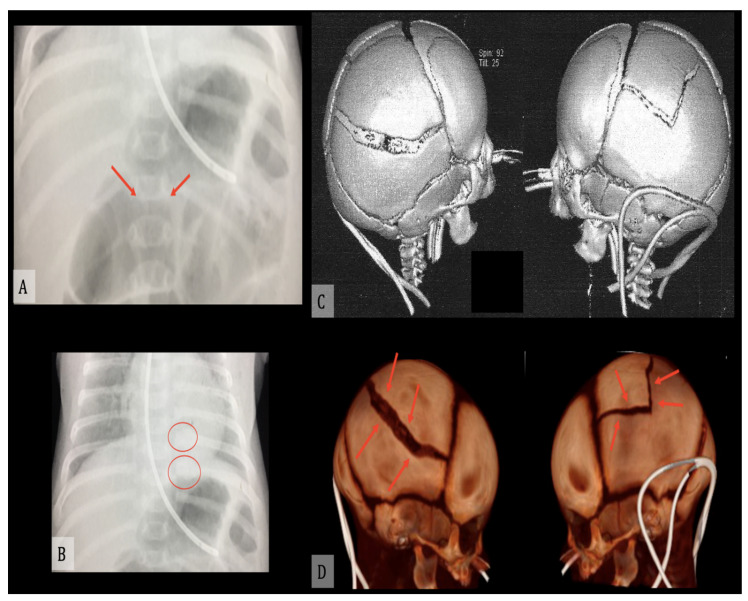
(A) Radiography of D11 soma fracture (red arrows). (B) Radiography of VII and VIII left rib fractures (red circles). (C,D) Three-dimensional CT reconstruction of skull fractures (red arrows).
